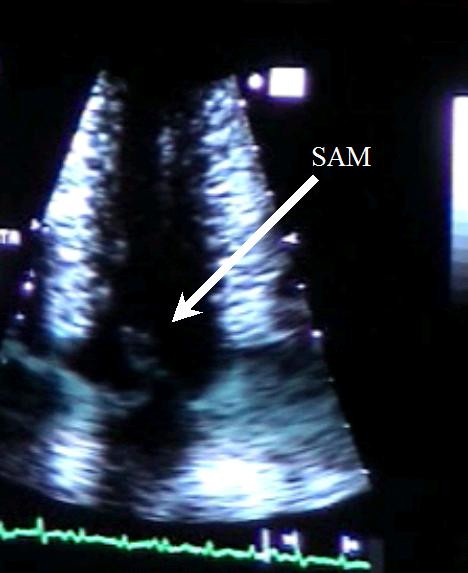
In this apical four chamber view obtained near peak exercise (before stoping) we can clearly see systolic anterior movement of mitral valve.
